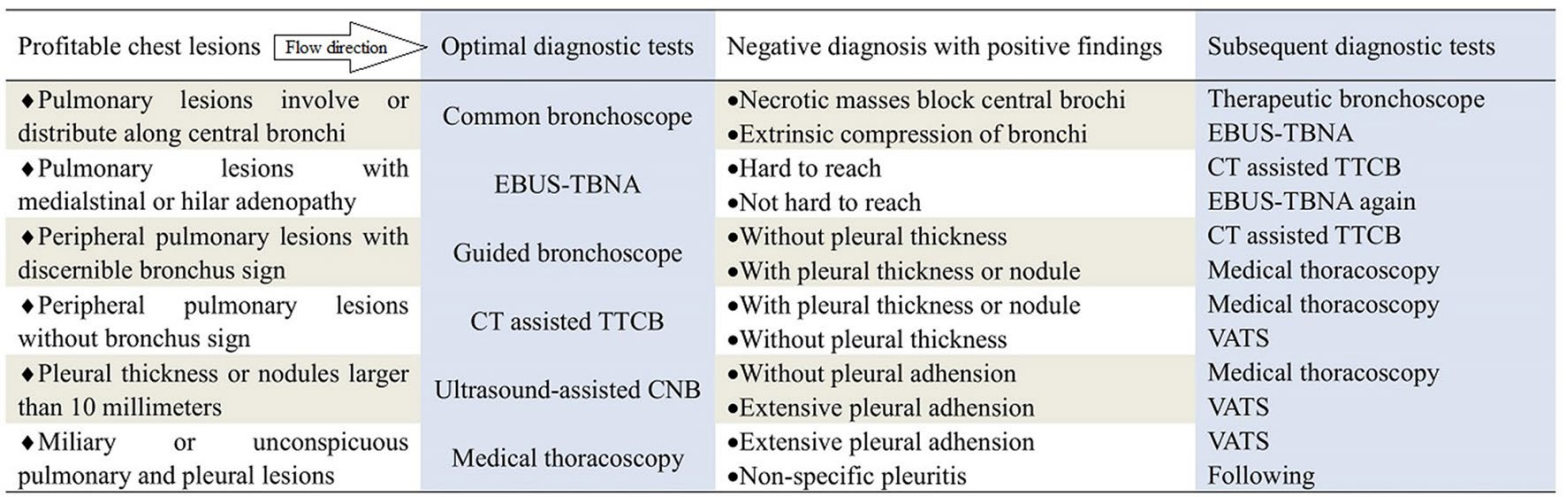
A clinical pathway to guide the selection of optimal and subsequent diagnostic tests. The optimal diagnostic tests in the first grey column were selected depending on the features of chest lesions in the corresponding line of the previous column. The subsequent diagnostic tests in the second grey column were selected depending on the findings of optimal diagnostic tests. *EBUS-TBNA* endobronchial ultrasound-guided transbronchial needle aspiration biopsy, *TTCB* transthoracic core biopsy, *CNB* cutting needle biopsy, *VATS* video-assisted thoracoscopic surgery.
